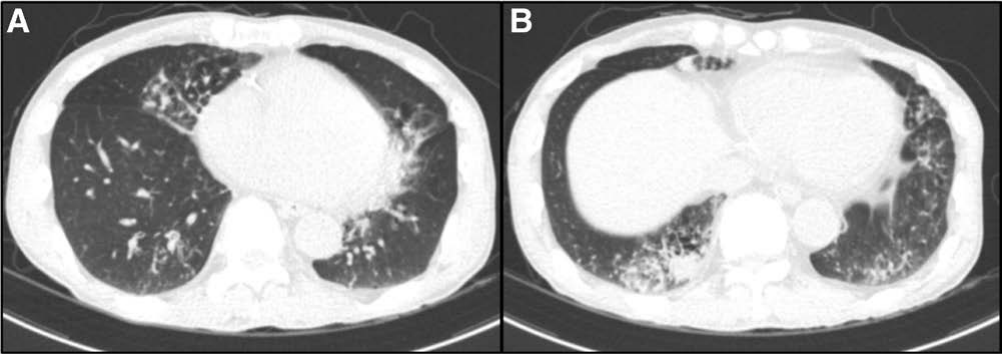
Initial chest computed tomography findings. (A and B) Chest CT showed bilateral and multifocal ground glass opacities and no other evidence of an enlarged lymph node or mass. Emphysema was found in both lung fields.
